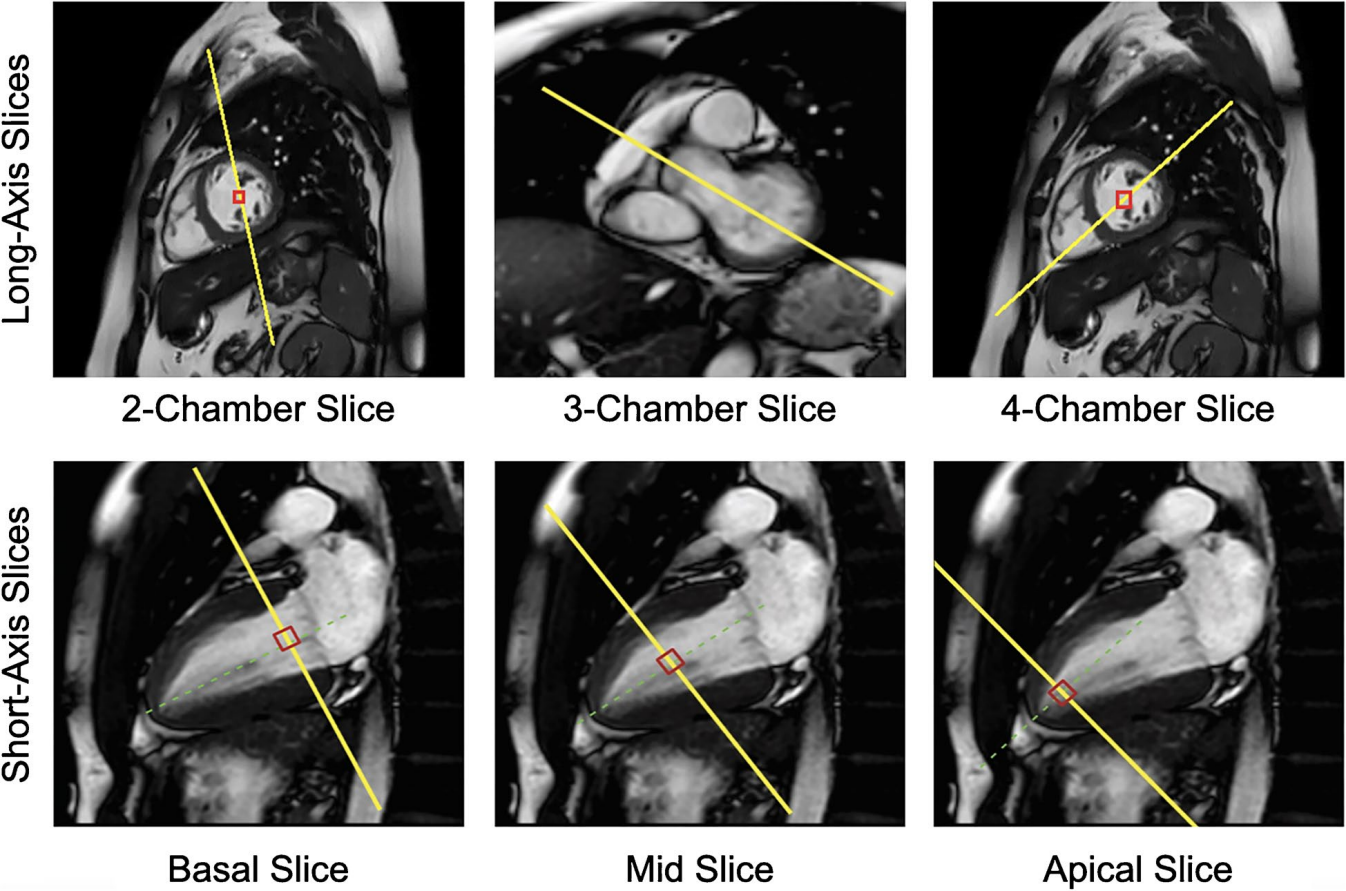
Fast-strain encoded (f-SENC) cardiac MRI protocol prescriptions. MyoHealth prescription in a 14-year-old male with Ewing sarcoma and anthracycline-induced cardiomyopathy. Six pre-contrast, separate fast-strain encoding acquisitions of the LV were performed at end systole. One of each: 2-chamber, 3-chamber, and 4-chamber slices, and three separate single short-axis slices were obtained at the base, mid, and apical levels. 2-Chamber: Prescribed off 4-chamber cine and mid short-axis stack. 3-Chamber: Prescribed off 2-chamber and basal short-axis cine. 4-Chamber: Prescribed off 2-chamber and a mid-short axis stack at end systole
